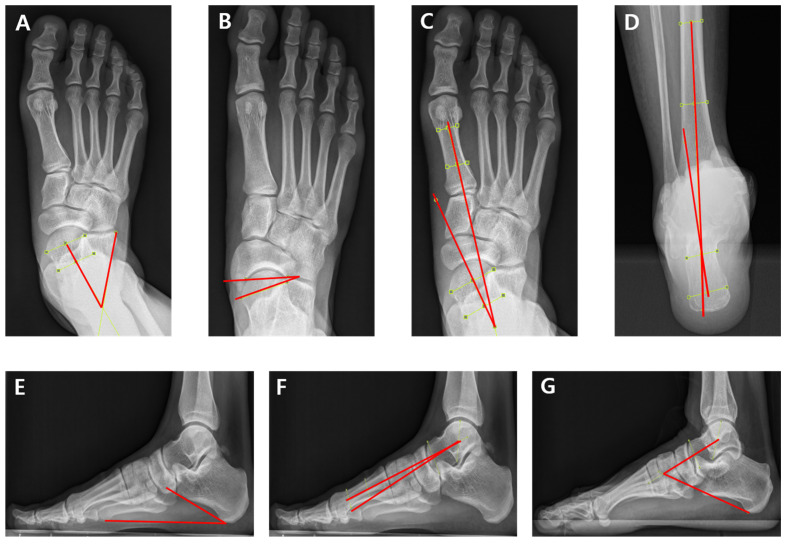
Radiographic measurements of the foot and ankle. (A) Anteroposterior talocalcaneal angle: the angle formed between a line bisecting the anterior surface of the talus and a line along the lateral border of the calcaneus, (B) talonavicular coverage angle: the angle between a line connecting the medial and lateral margins of the anterior talar articular surface and a line connecting the medial and lateral margins of the proximal navicular articular surface, (C) anteroposterior talo-first metatarsal angle: the angle between a line bisecting the anterior surface of the talus and the longitudinal axis of the first metatarsal, (D) hindfoot alignment angle: the angle formed by the long axis of tibia and the long axis of the calcaneus, (E) calcaneal pitch angle: the angle between a line drawn along the edge of the plantar soft tissue shadow and a line along the inferior border of the calcaneus, (F) Meary’s angle: the angle between the longitudinal axis of the talar head and the first metatarsal bone, and (G) lateral talocalcaneal angle: the angle formed by the long axis of the talus and a line along the inferior border of the calcaneus.
